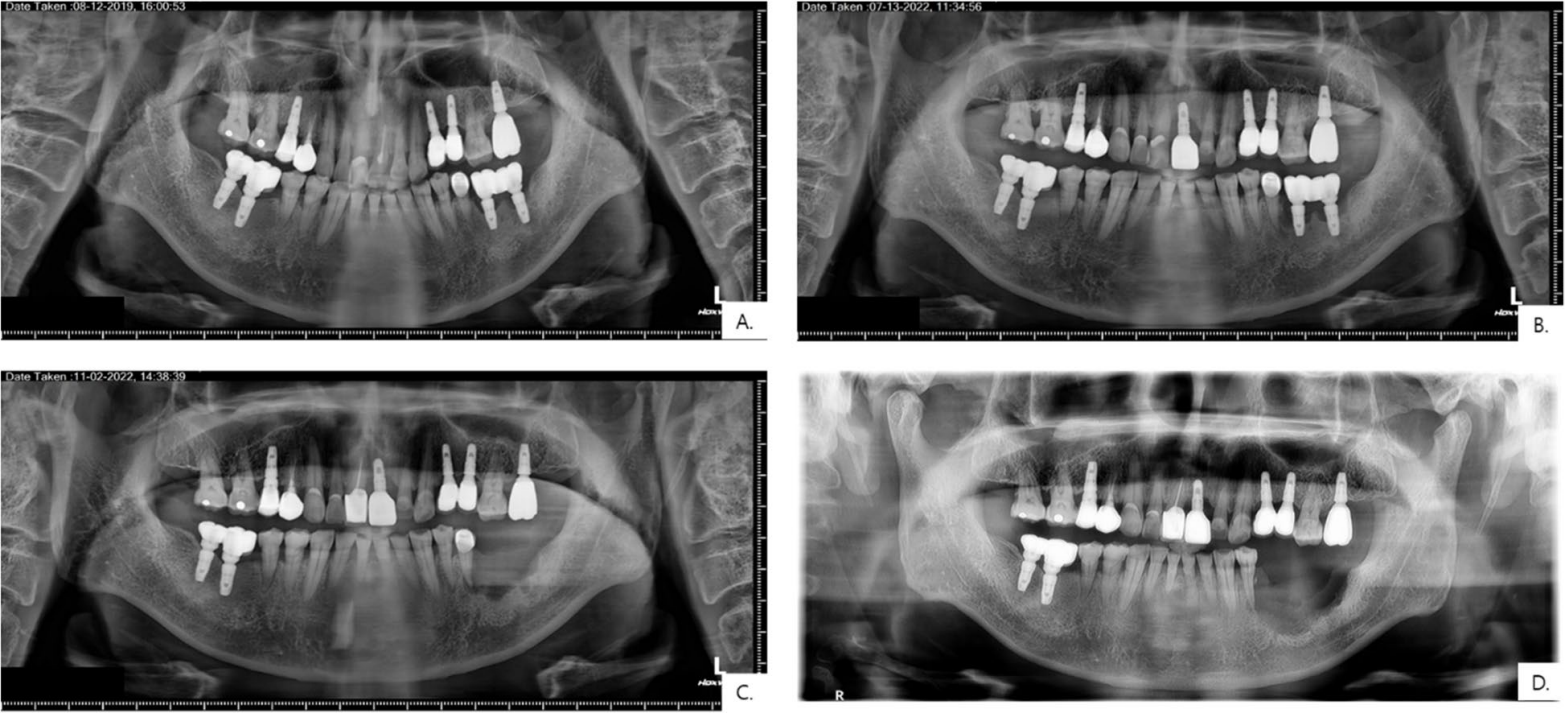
Serial panoramic radiographs of a patient before OSCC diagnosis. The patient underwent implant placement at the left mandible (#36, 37) in 2008 at a local clinic. A August 2019: marginal bone loss noted around #36, 37. (panoramic X-ray from the local clinic). B July 2022: progressive bone loss observed with discomfort (panoramic X-ray taken from the local clinic). C November 2022 (after implant removal): further bone loss encroaching #35. (panoramic X-ray from the local clinic). D February 2023 (Department of Oral and Maxillofacial Surgery): severe bone destruction; incisional biopsy confirmed OSCC following referral from the local clinic
